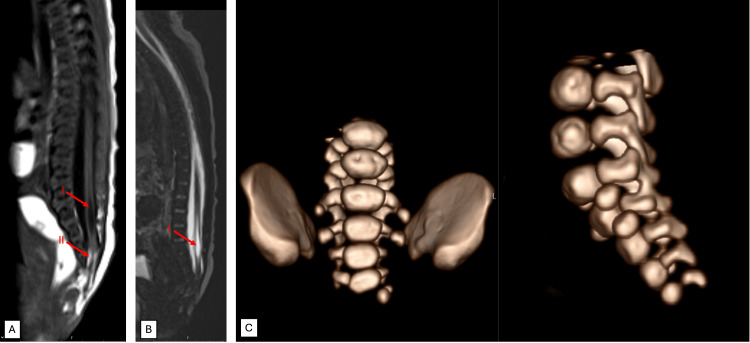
MRI (A and B) and CT (C) findings showing tethered cord, intraspinal lipoma, hydromyelia, and sacral partial agenesis. (A) T1-weighted sagittal magnetic resonance imaging (MRI) showing mild caudal regression with a tethered cord, evidenced by the abnormally low position of the conus medullaris below the L1-L2 level. Additional findings include syringohydromyelia (I) and an intraspinal terminal lipoma contiguous with subcutaneous fat (II). (B) Volumetric 3D T2-weighted sagittal MRI sequence in the sagittal plane demonstrating the tethered cord and syringohydromyelia (I). (C) Volumetric reconstruction of computed tomography (CT) of the lumbosacral spine showing partial agenesis of S5 and agenesis of the coccyx. Note the presence of movement artifacts. These artifacts are inherent challenges in neonatal imaging and may slightly affect the resolution of certain details.
